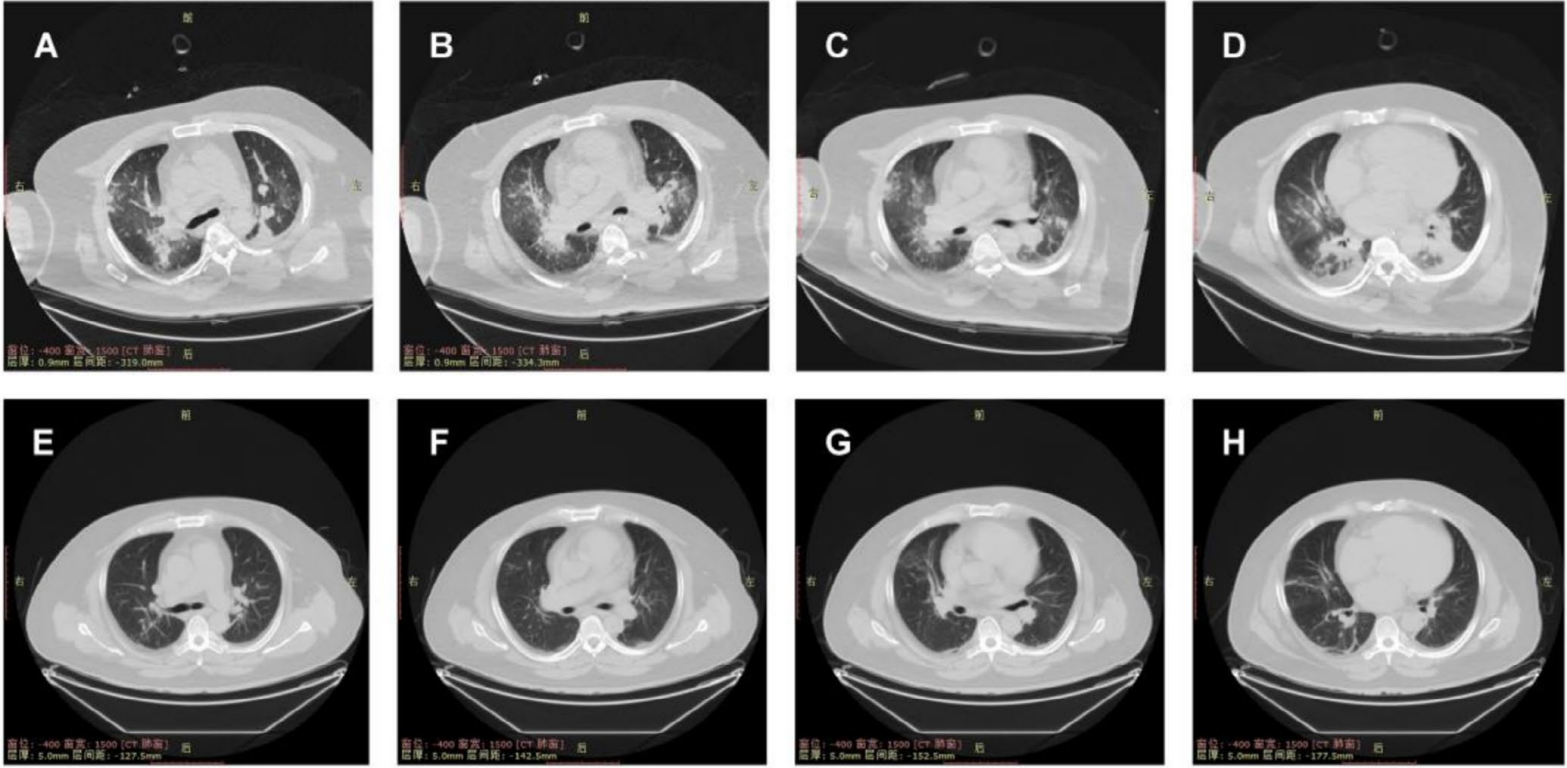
Chest CT on day 1 (A–D) and day 9 of admission (E–H).
